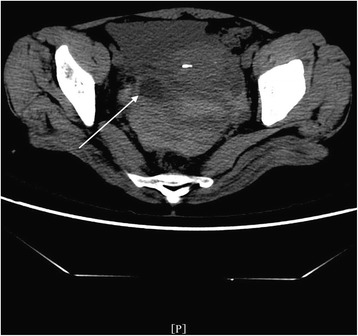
CT findings of REPWH. The patient had a history of amenorrhea for 54 days and a positive HCG test. Right ovarian ectopic pregnancy rupture was confirmed by surgery. The single arrow indicates a cystic shadow (18 mm × 19 mm) on the right side of the adnexal area
